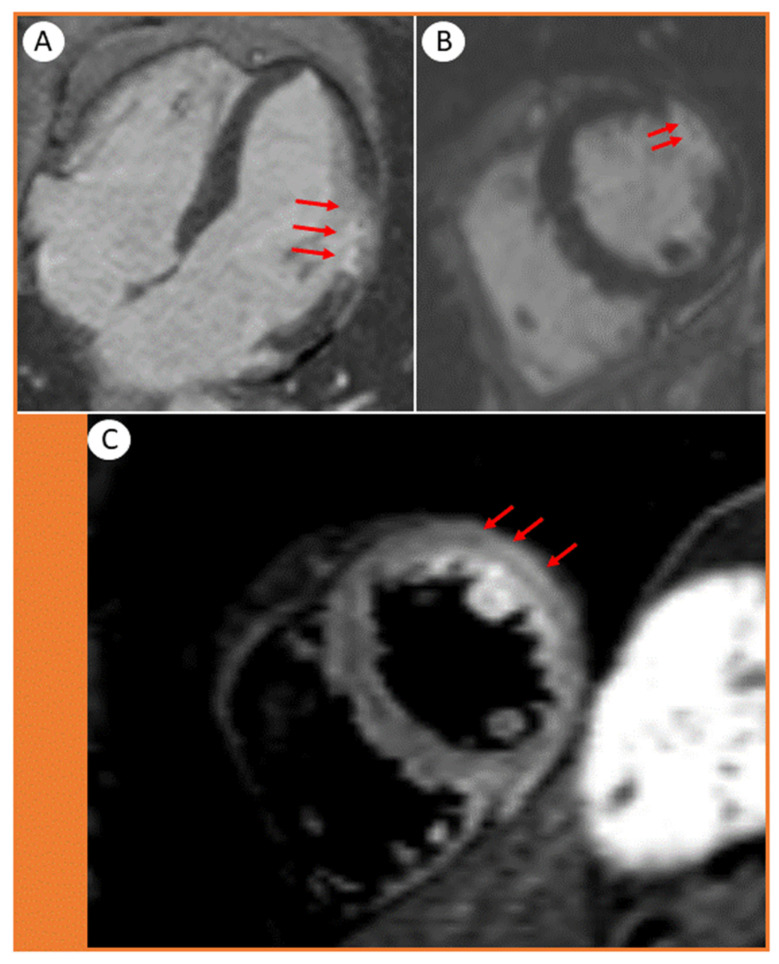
Cardiac magnetic resonance images showing (A) 4-chamber and (B,C) short axis views. The transmural distribution of late gadolinium enhancement (LGE) in the lateral wall of the left ventricle displays the extent of the infarction. (B, arrows) Microvascular obstructions are presented as tiny spots within the LGE. (C, arrows) The short-axis STIR acquisition shows edema in the lateral wall and papillary muscle.
